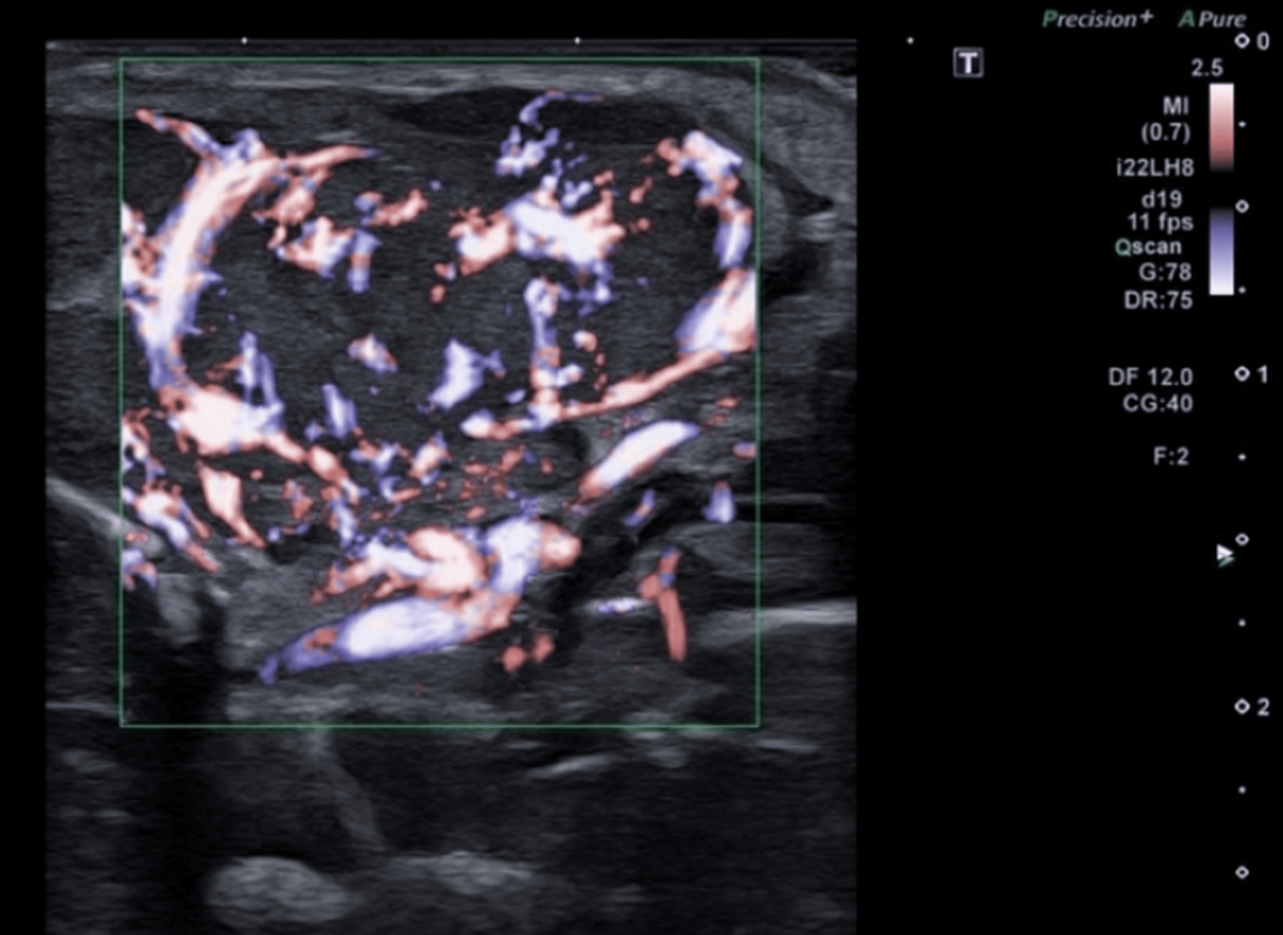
Doppler imaging of the lesion shows intense, diffuse vascularization.
